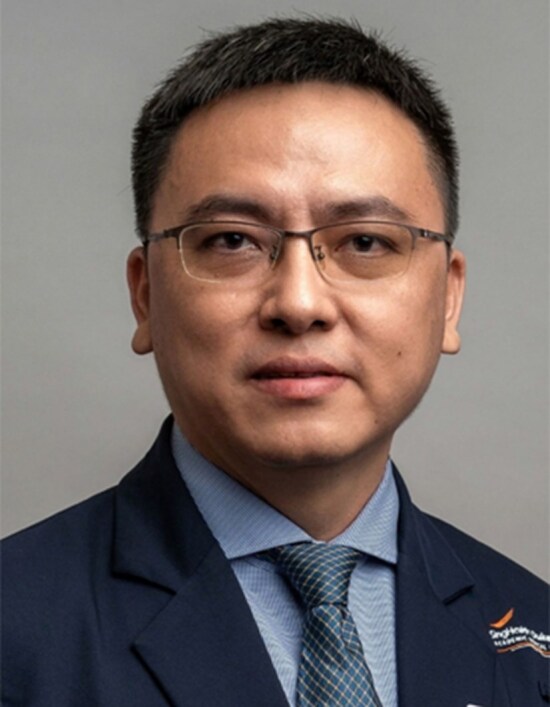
Dr Shuang Leng is affiliated with the National Heart Centre Singapore and Duke-NUS Medical School Singapore. His expertise lies in cardiac image processing and computer science, with research interests spanning the analysis of cardiac MR, CT, and 3D echocardiographic images. He focuses on algorithm development for cardiac segmentation and the quantification of regional contractile function and myocardial strain, as well as the application of artificial intelligence in cardiovascular image analysis.
